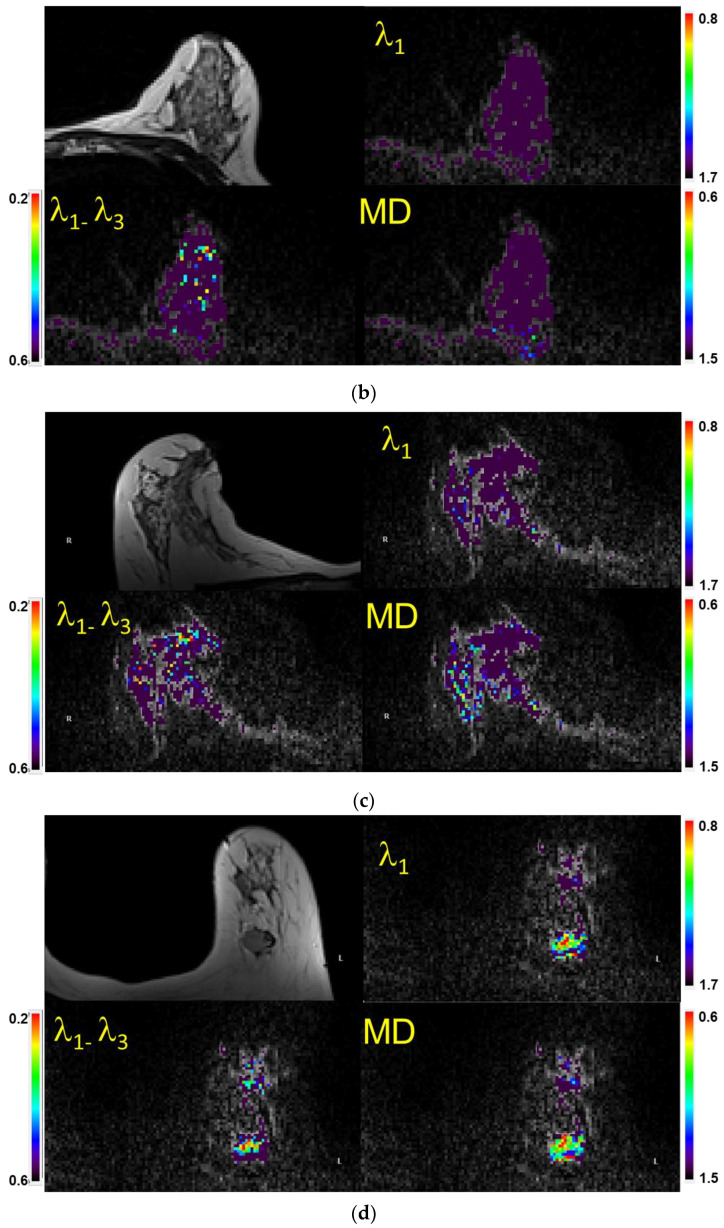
(a) Example of a case with a lesion (mass) identified (visual score = 2) on a fatsat T2-weighted MRI (top left), characterized as benign semi-quantitatively, and confirmed quantitatively. Shown are the λ1 map, MD/ADC map, and maximal anisotropy (λ1–λ3) map. Note that the color pixels in the mass are uniformly purple on the λ1 and MD maps, confirming that the lesion is benign (Bx score = 0). Recommendation: No biopsy. Subsequent biopsy confirmed a benign fibroadenoma. The values of λ1, MD, and λ1–λ3 in the corresponding scales are ×10^−3^ mm^2^/s. Arrows indicate the lesion. (b) Example of a case with no lesion identified on MRI (visual score = 0) and in which=ch a biopsy was not indicated. When no lesion could be identified, the readers used the fact that there were no visible non-purple pixels within the breast parenchyma in the expected region of the lesion to score the case as benign (Bx score = 0). Shown are the T2-weighted image (Top left), λ1 map, MD/ADC map, and maximal anisotropy (λ1–λ3) map overlaid on the corresponding B0 image. Recommendation: No biopsy. The values of λ1, MD, and λ1–λ3 in the corresponding scales are ×10^−3^ mm^2^/s. (c) Example of a case with no lesion identified on MRI (visual score = 0). When no lesion could be identified and there were multiple non-purple pixels within the breast parenchyma in the area of interest, the scan was scored as indeterminate (Bx score = 1). Recommendation: Proceed with biopsy. Shown are atheT2-weighted image (Top left), λ1 map, MD/ADC map, and (λ1–λ3) map overlaid on the corresponding B0 image. The random distribution of non-purple pixels is a result of limited signal to noise in the diffusion-weighted images. The values of λ1, MD, and λ1–λ3 in the corresponding scales are ×10^−3^ mm^2^/s. (d) Example of a case with a mass identified (vis. score = 2) on a fatsat T2-weighted MRI (top left) and characterized as malignant both semi-quantitatively and quantitatively (Bx score = 2). Shown are the λ1 map, MD/ADC map, and anisotropy (λ1–λ3) map overlaid on the corresponding B0 image. Note the non-purple color pixels in the mass. Recommendation: Biopsy. Subsequent biopsy confirmed an invasive ductal carcinoma (IDC). The values of λ1, MD, and λ1–λ3 in the corresponding scales are ×10^−3^ mm^2^/s.
